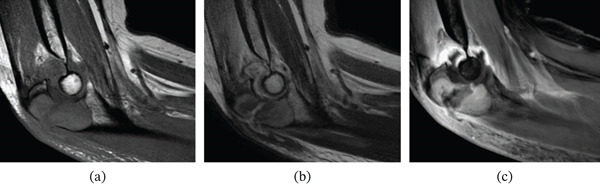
Preoperative sagittal magnetic resonance imaging of the olecranon. (a) T1‐weighted image. (b) T2‐weighted image. (c) Gadolinium‐enhanced T1‐weighted fat‐suppressed image demonstrating an intraosseous lesion without extraosseous extension.
